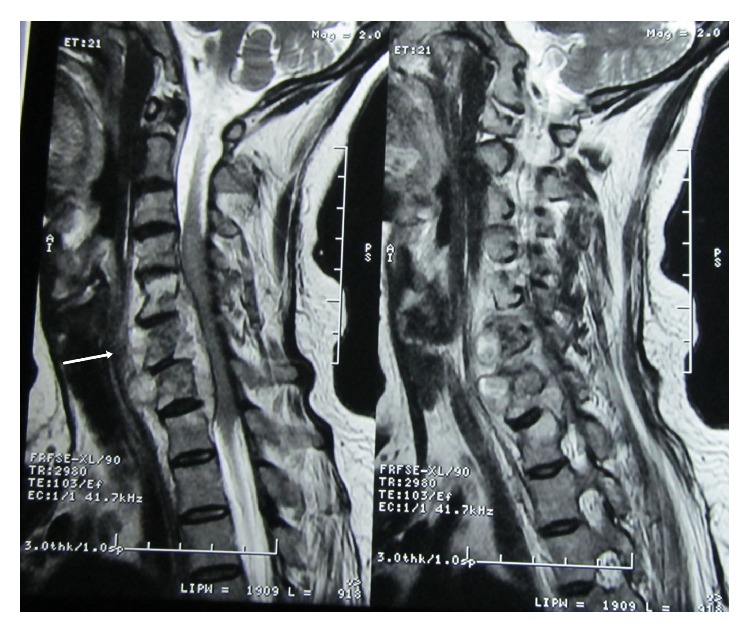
MRI of cervicodorsal spine showing altered marrow signal intensity with erosion of C5 to D1 vertebrae and compression of spinal cord.
